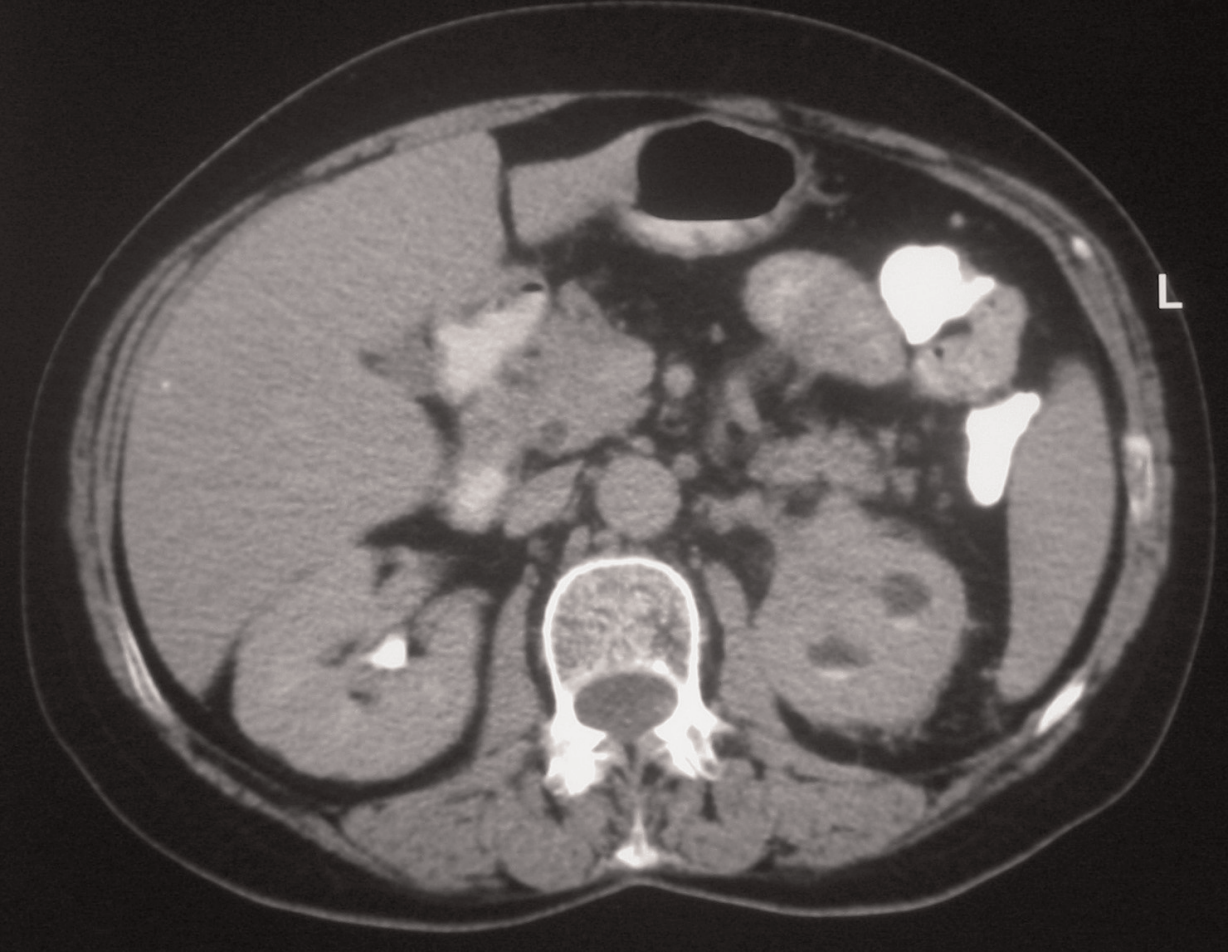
Axial computed tomography scan revealing faint excretion of contrast medium in the lower pole calyces.
